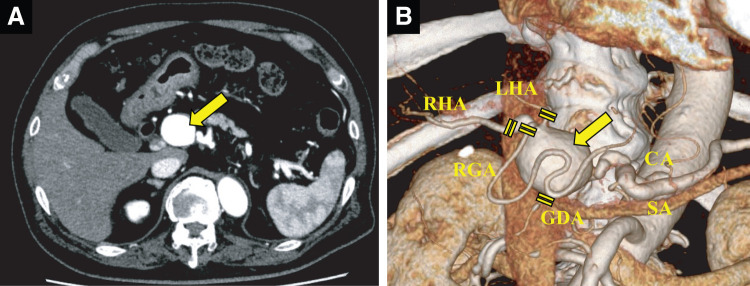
Enhanced CT showed a large aneurysm. (A) A large aneurysm (large yellow arrow) located at the CHA-PHA. (B) 3D imaging of the abdominal artery from the CA. Four outflow arteries from this aneurysm (large yellow arrow) were encircled and ligated/clipped. CA, celiac axis; CHA-PHA, common-proper hepatic artery; GDA, gastroduodenal artery; LHA, left hepatic artery; RGA, right gastric artery; RHA, right hepatic artery; SA, splenic artery
